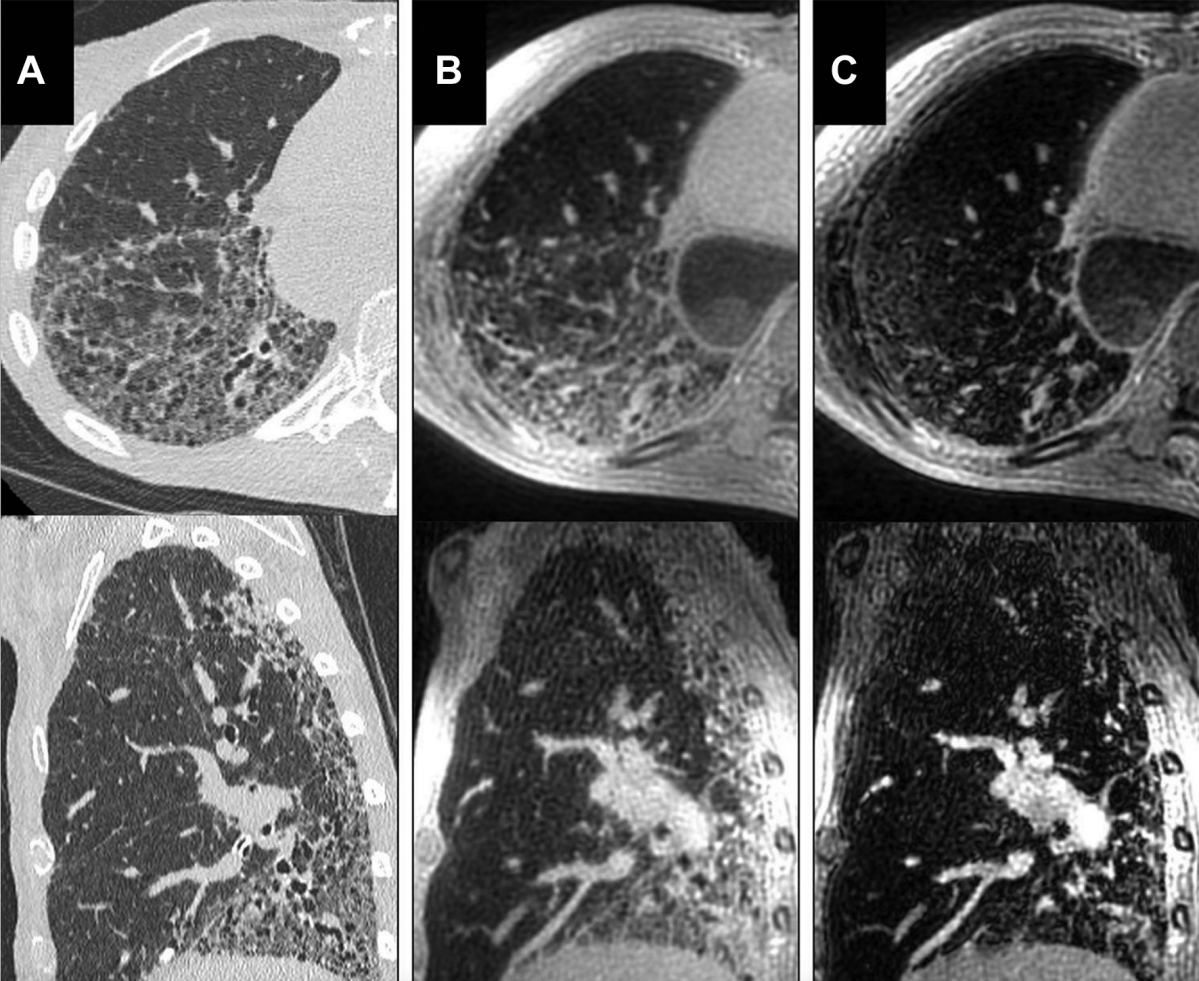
Axial and sagittal reformats of the high-resolution CT (HRCT) (A), three-dimensional ultrashort echo time (3D UTE) (B) and the second echo of the 3D UTE sequence (C). Although honeycomb change is visualised in the UTE image, HRCT images show higher spatial resolution. The structural abnormality is not well visualised in the second echo images. Reproduced with permission from John Wiley and Sons.^49^
